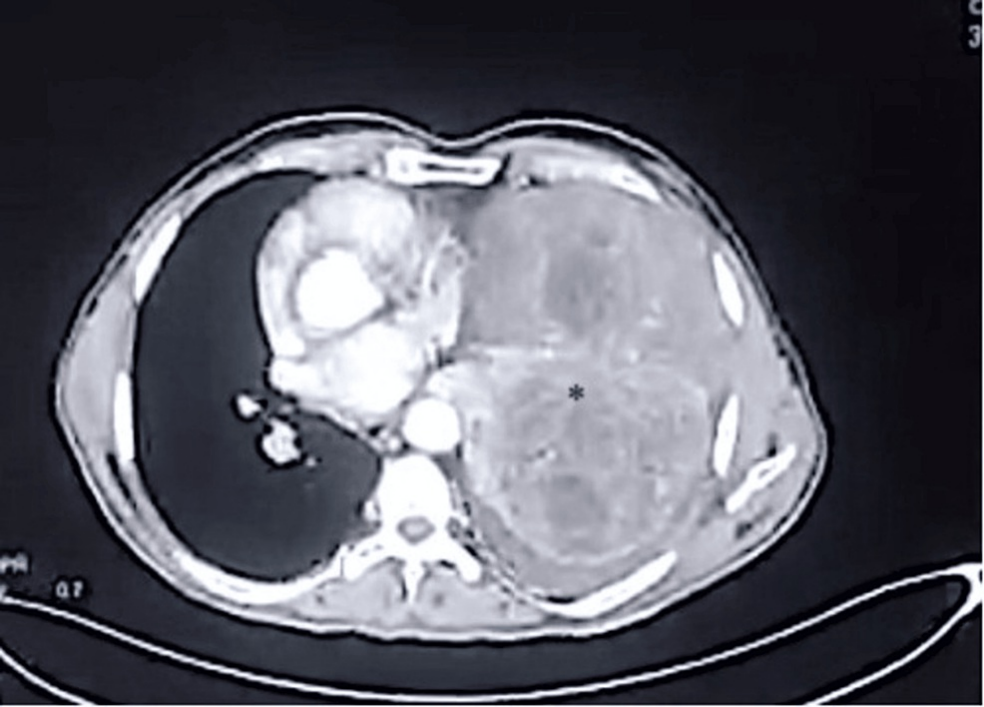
Contrast-enhanced computed tomography of the thorax shows a heterogeneously enhancing mass lesion in the left lung parenchyma, almost entirely replacing the left lung parenchyma (highlighted by asterisk).
